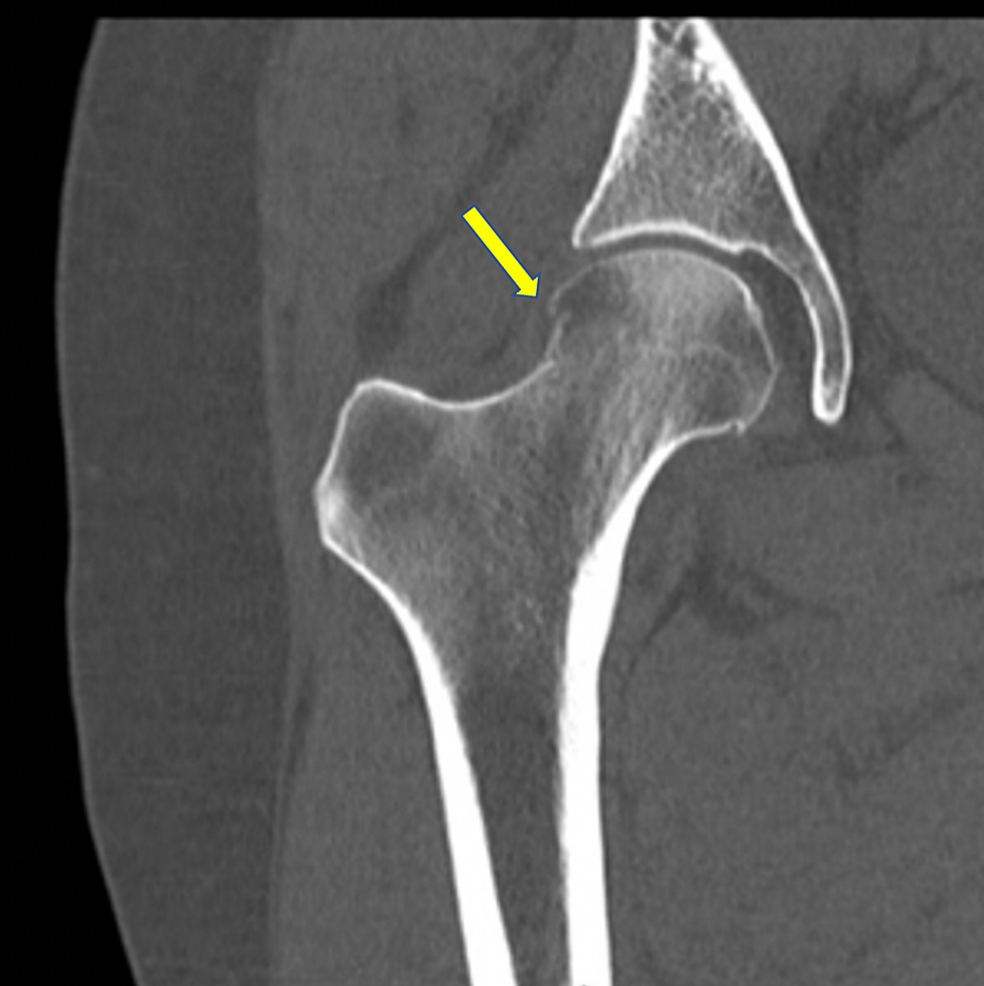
CT of the right hip. Cortical step-off (yellow arrow) suspicious for impaction-type fracture in the anterior aspect of the femoral head.
